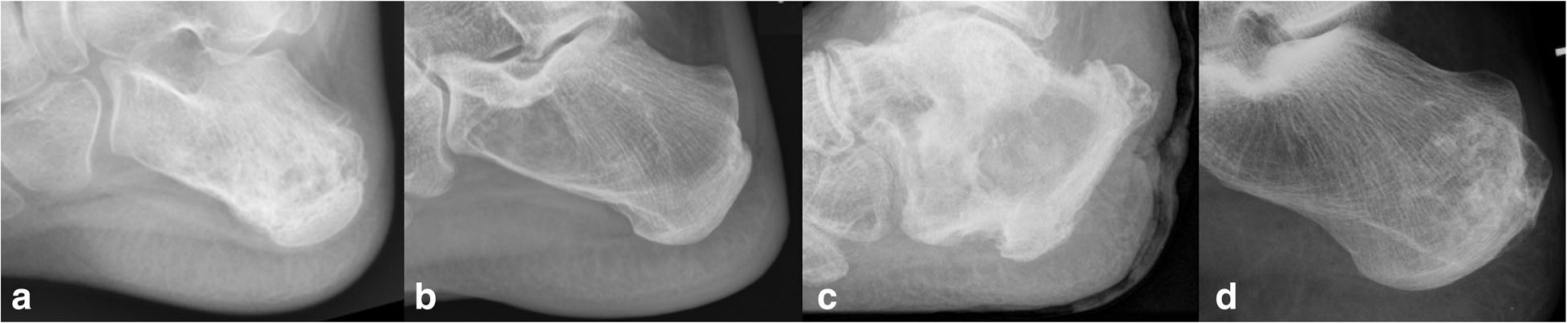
Osteolytic lesions of the calcaneus with different radiographic appearance and varying aggressive behaviour: (a) Ewing sarcoma in a 31-year old male patient, (b) simple (calcaneal) bone cyst in a 11-year old male patient, (c) secondary squamous cell carcinoma based on chronic osteomyelitis in a 82-year old male patient and (d) low-grade chondrosarcoma in a 45-year old female
